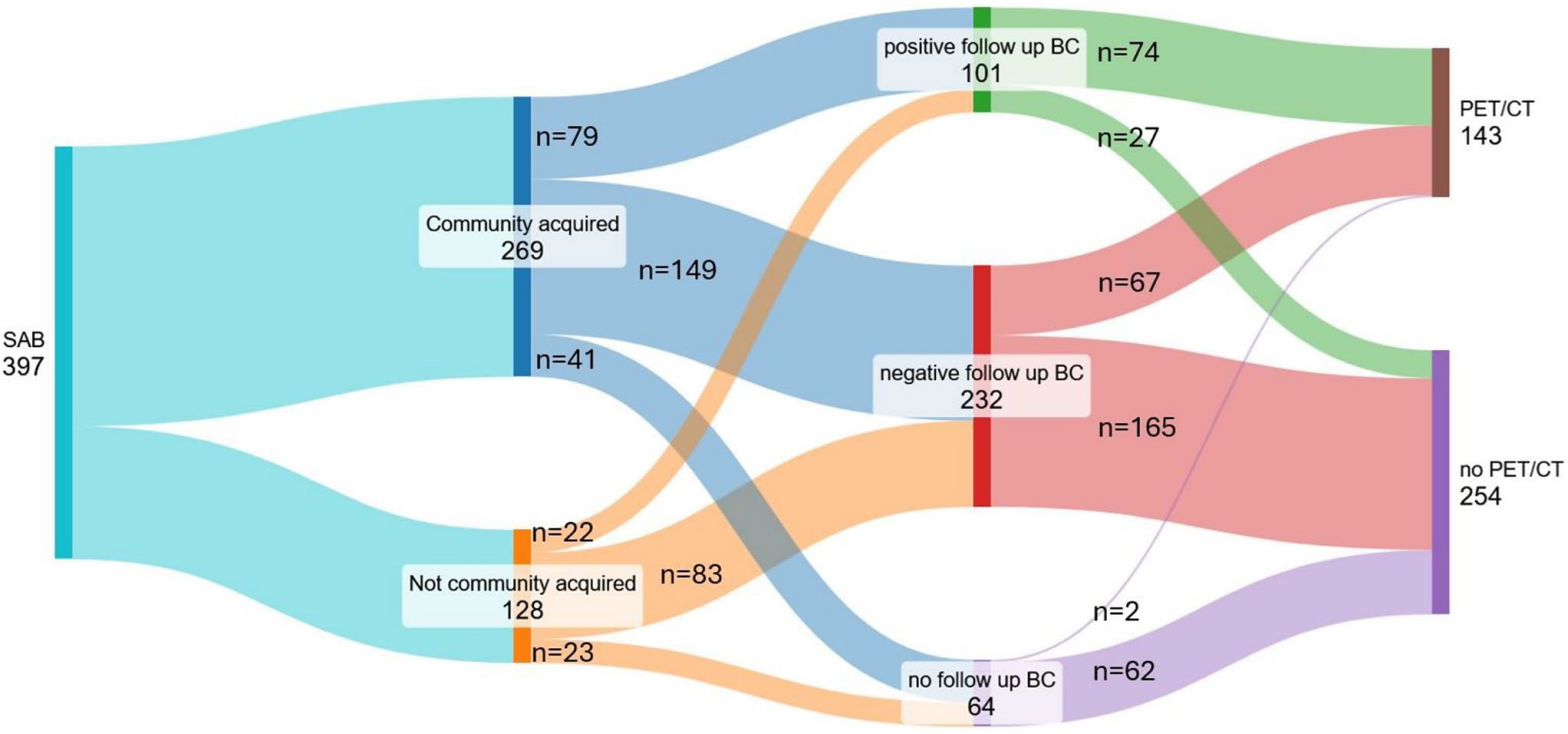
PET/CT performed in SAB patients according to origin and 48 h positive follow-up blood cultures. Legend: SAB: *Staphylococcus aureus* bacteremia; BC: blood cultures; follow up BC: Blood culture taken after approximately 48 h after the 1st positive BC and start of treatment
